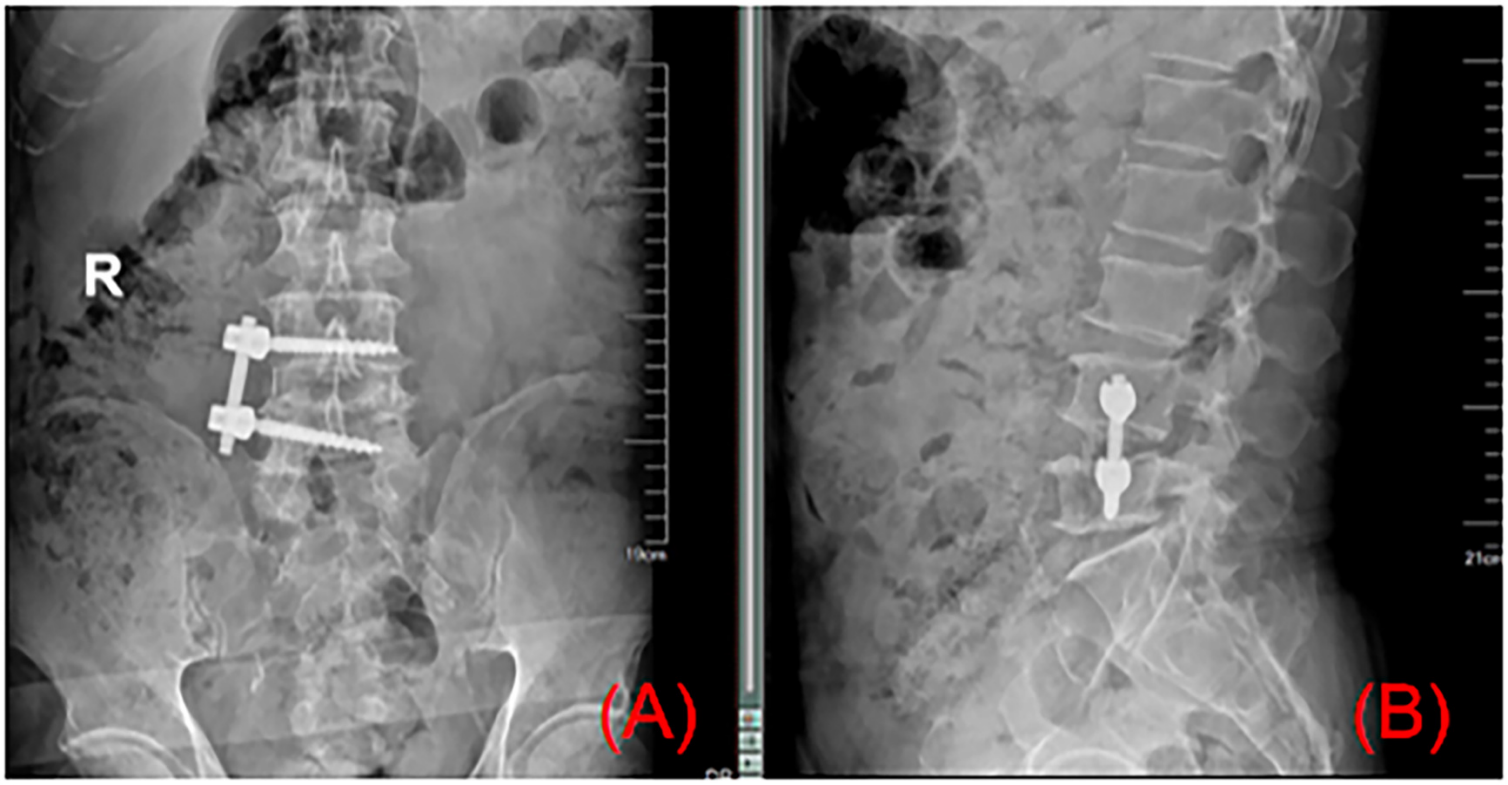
Postoperative radiographs (A,B) confirmed optimal positioning of the lateral screw-rod construct and interbody cage, consistent with intraoperative fluoroscopic findings.
